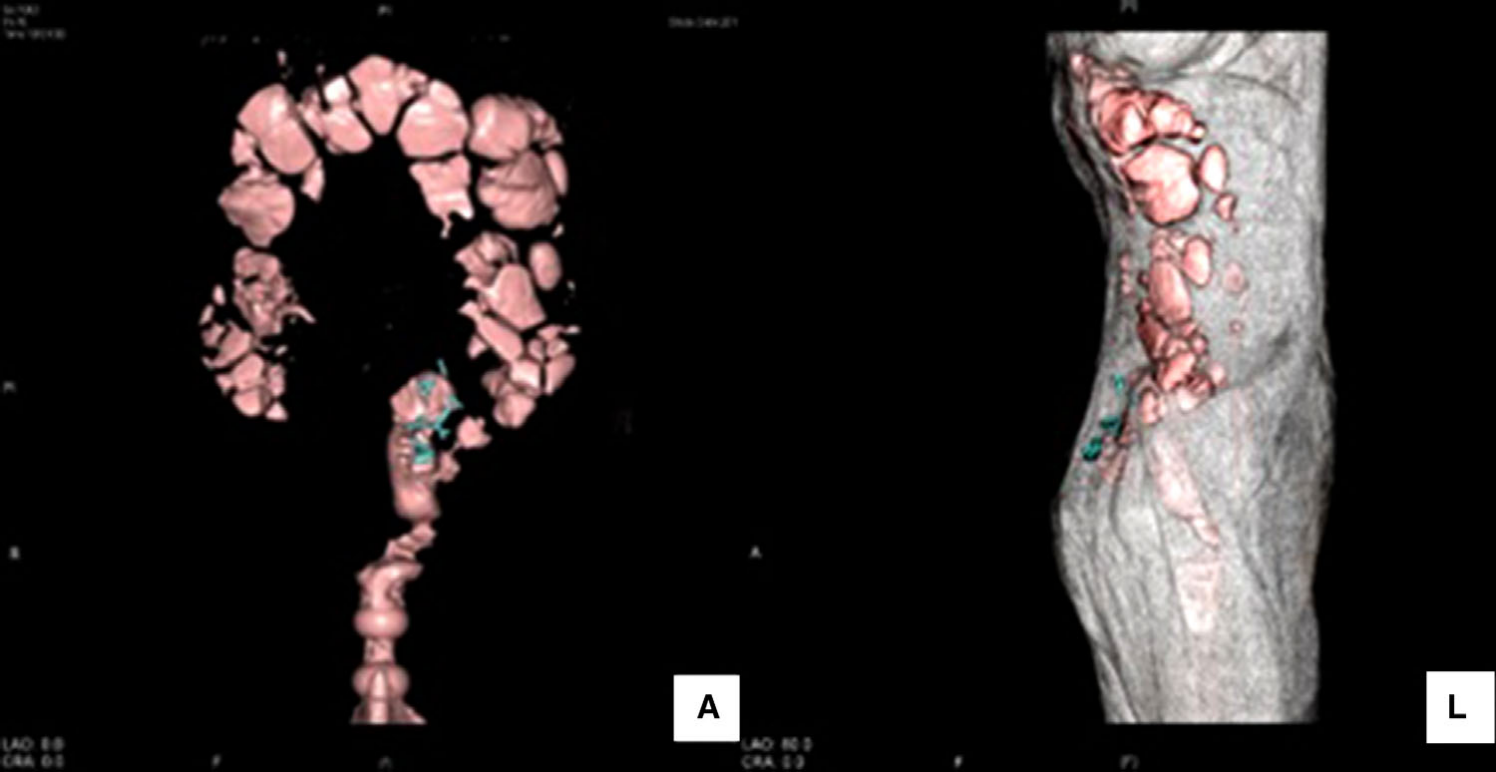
Computed tomography scan taken 5 years after the colonoscopy‐assisted percutaneous sigmoidopexy: T‐bars of the two‐shot anchor that fix the sigmoid colon and the rectum to the abdominal wall at a straightened angle. No recurrence was observed
